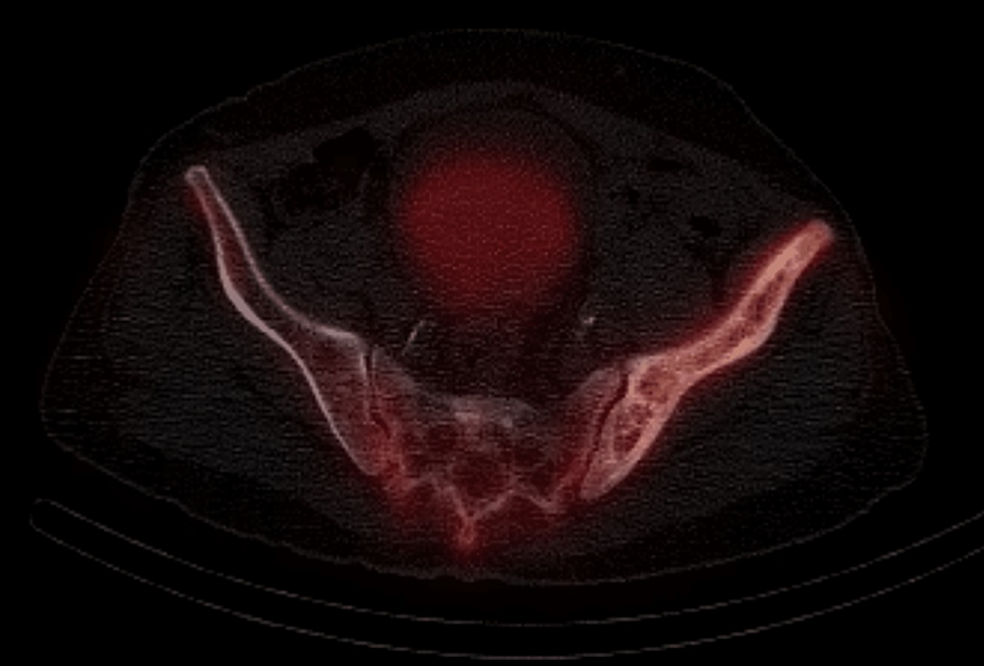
Selected axial SPECT-CT images showed heterogeneous sclerosis, cortical thickening, and mild bone expansion of the left hemipelvis with moderate to marked increases in radiotracer uptake
